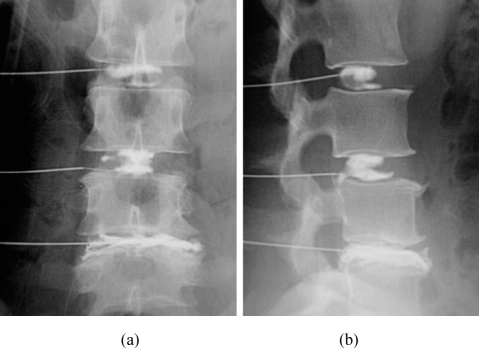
Discographic patterns in a 43-year-old woman who had low back pain with radiation to the left calf. (a) Anteroposterior and (b) lateral radiographic projections show a normal bilocular L2/3 disc. There is small posteroinferior tear of the L3/4 disc that was asymptomatic. The L4/5 disc is decreased in height, and had extensive annular disruption and posterior protrusion. The L4/5 disc was also symptomatic.
